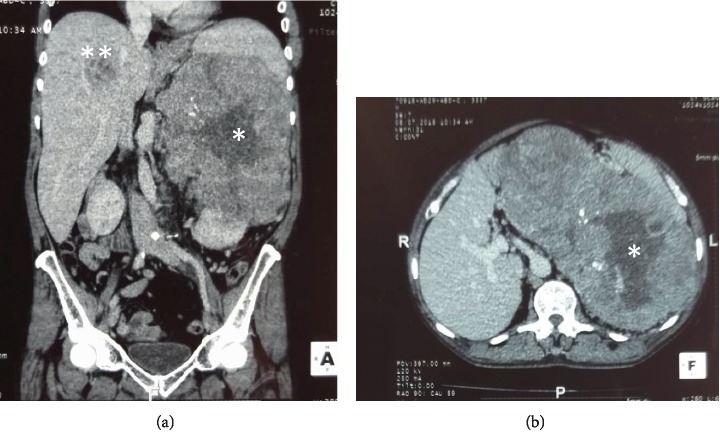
Contrast-enhanced CT abdomen, chest, and pelvis: (a) coronal image and (b) axial image showing heterogeneously enhancing left suprarenal mass (21 × 18 × 24 cm)^*∗*^ with central necrosis and foci of calcifications. Focal liver metastasis^*∗∗*^ is also seen.
